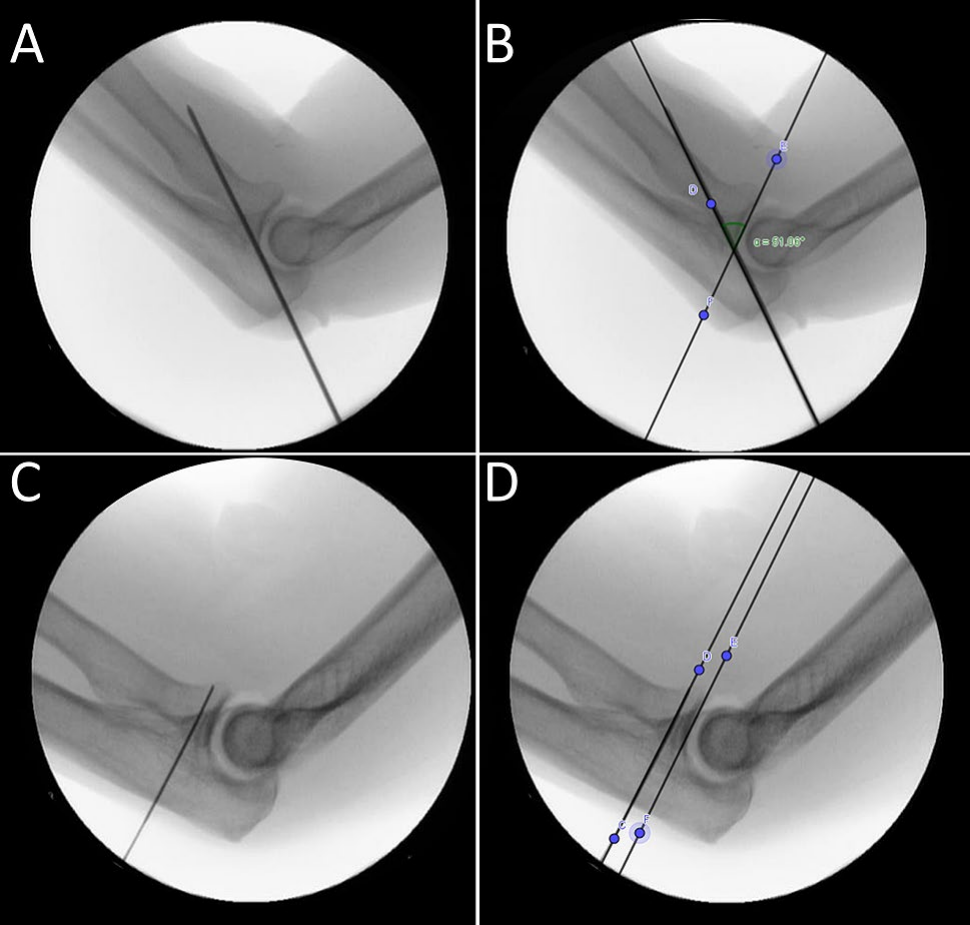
Radiographs after placement of the guiding k-wire with the corresponding measurement of the inclination angle α. Radiograph after free-hand placement of the guiding k-wire in the radial head (A) showing an inclination angle of 51° (B), and radiograph after using a reference k-wire in the radiocapitellar joint without parallel k-wire guide (C) and an almost parallel guide k-wire in the radial head (D)
